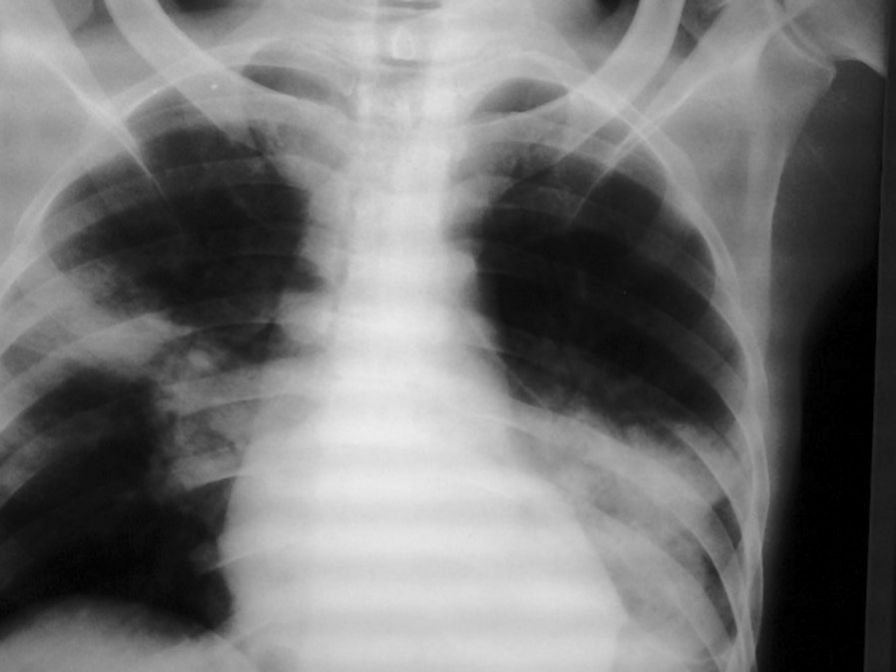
Chest X-ray of NN showing bilateral opacities
